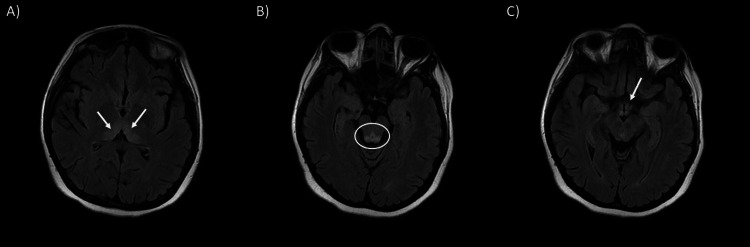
Axial T2 FLAIR images showing an increased signal in the (A) bilateral dorsal thalami, (B) periaqueductal white matter and (C) bilateral mammillary bodies FLAIR, fluid-attenuated inversion recovery
